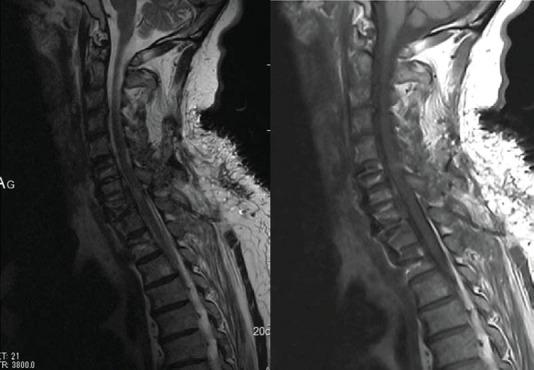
MRI postoperative does not show any abnormality of the spine canal.
